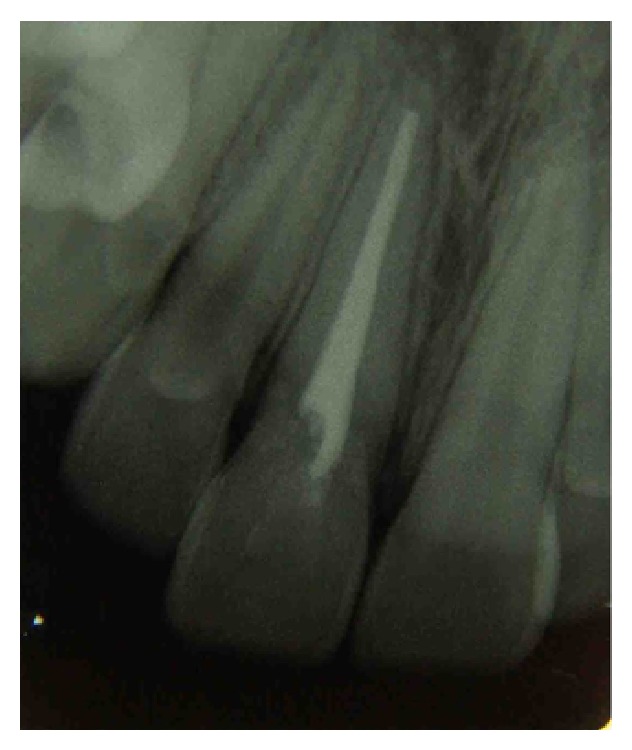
Radiograph after endodontic treatment.
